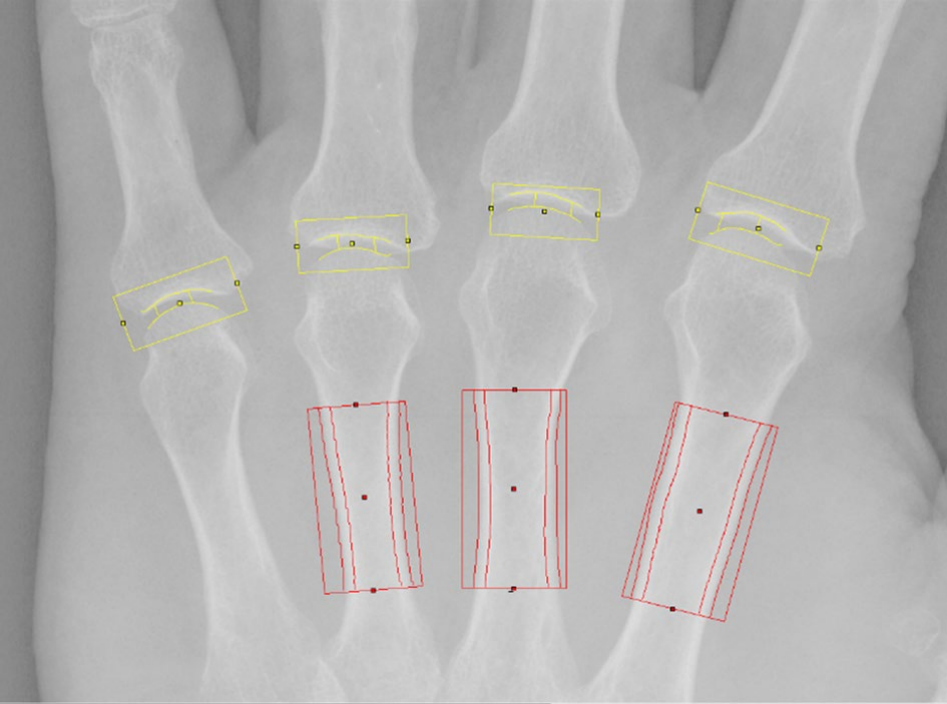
Computer-based structural analysis using digital X-ray radiogrammetry (DXR) for the quantification of metacarpal bone mineralization (region of interest: red box) and computer-aided joint space analysis (CAJSA) for the quantification of finger joint space distance (region of interest: yellow box).
